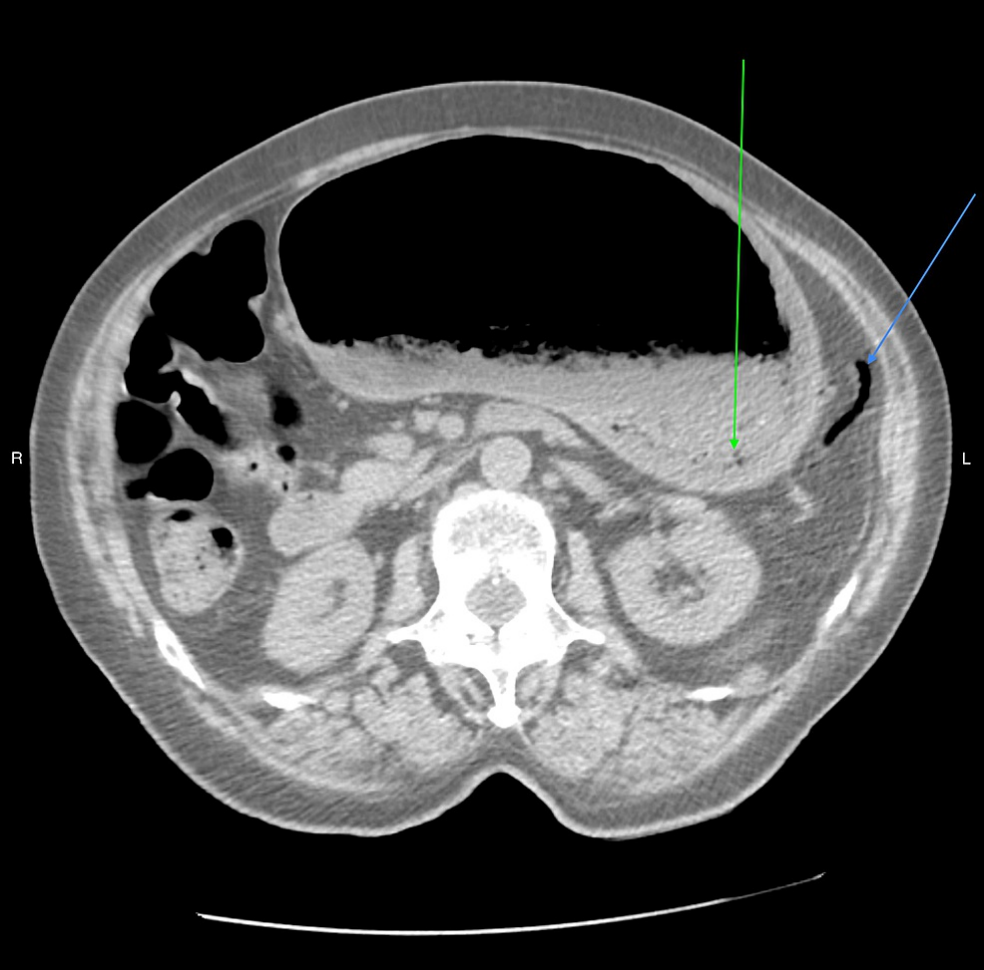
CT scan of abdomen without contrast (axial view) demonstrating distended stomach, thickened gastric wall with gas bubbles (green arrow), and gas in peri-gastric vein along the greater curvature of stomach (blue arrow).
